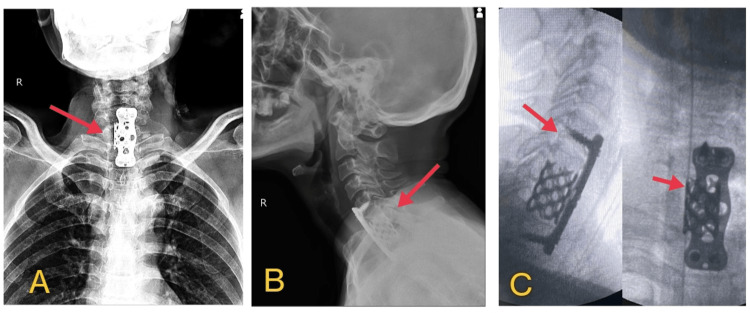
Radiographic images with arrows showing anterior cervical spine instrumentation with plates and screws A. X-ray PA view (posteroanterior view) B. X-ray lateral view C. X-ray showing plates and screws.
